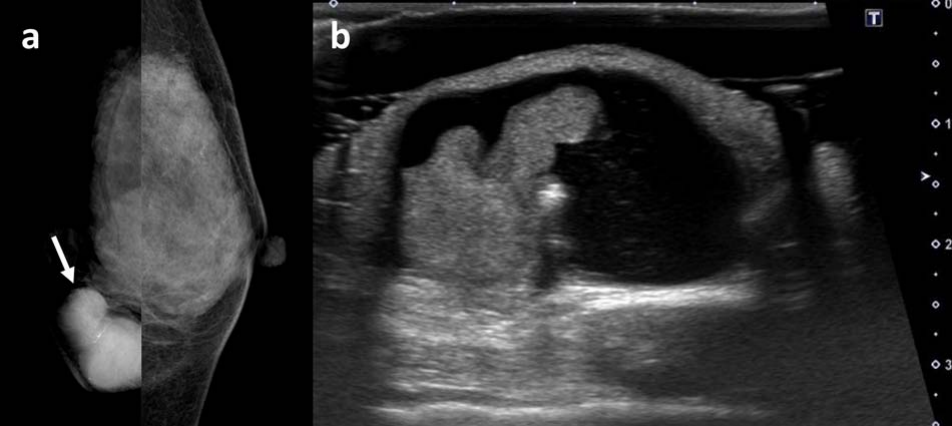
Clinical images of the right breast tumour in 2020. (a) Mammography showing a high-density mass with circumscribed margins (arrow). (b) Ultrasonography showing a 38-mm complicated cyst.
